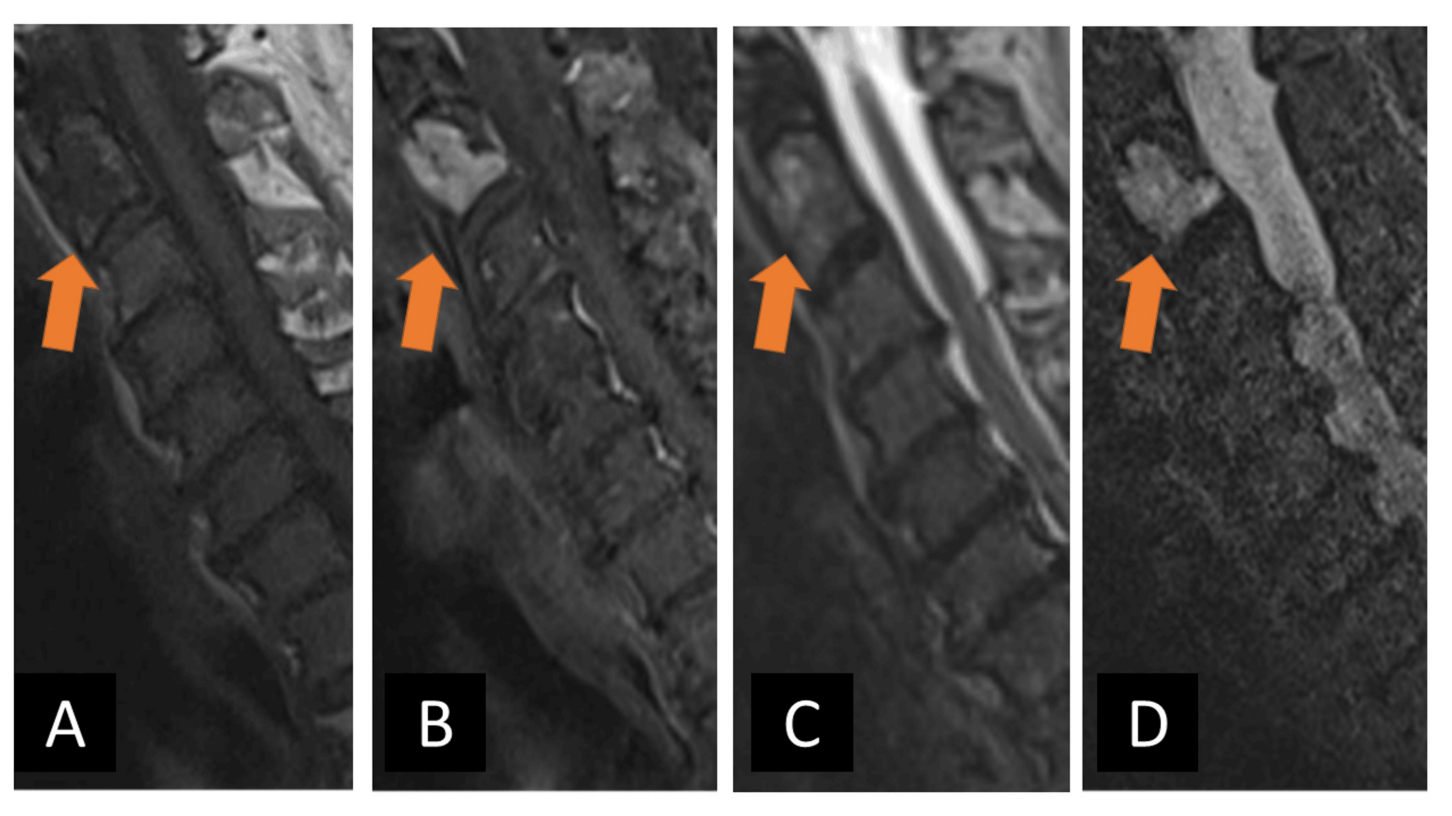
Cervical spine MRI shows a C2 lesion with anterior extraosseous extension (arrows). Cervical spine MRI shows a C2 lesion with anterior extraosseous extension (arrows) exhibiting A) low signal on non-contrast T1 weighted sequence, B) homogeneous contrast enhancement on post-contrast fat-saturated T1-weighted sequence, C) nearly isointense T2 signal, and D) hyperintense T2 STIR signal. There is no evidence of pathologic fracture. STIR: Short Tau Inversion Recovery
